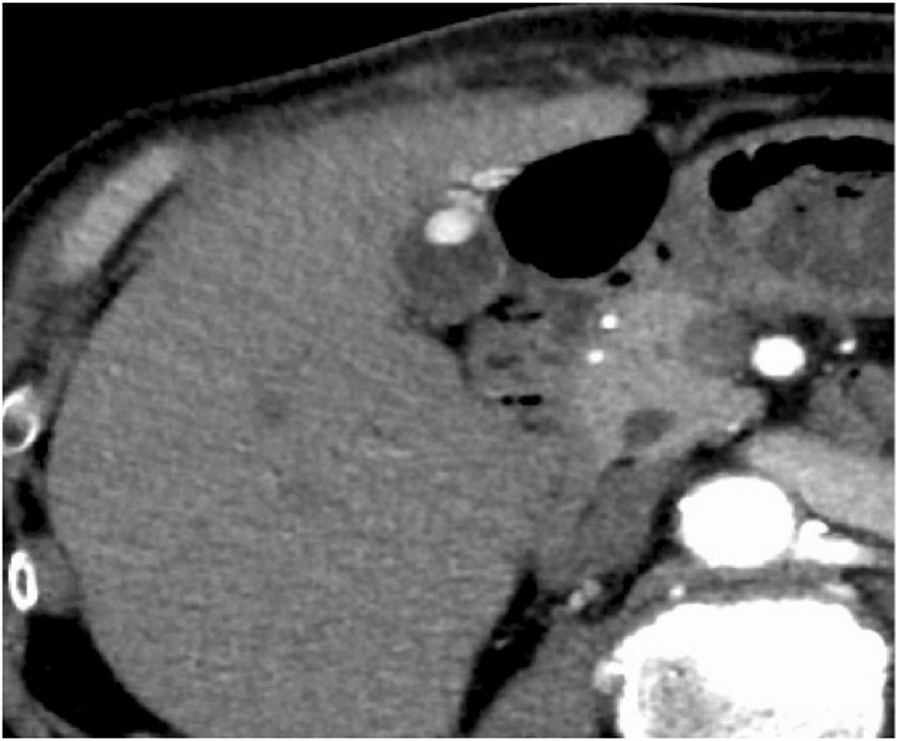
Dynamic CT scan showed a polypoid mass of the gallbladder. In arterial phase, it revealed very high and homogeneous contrast effect
